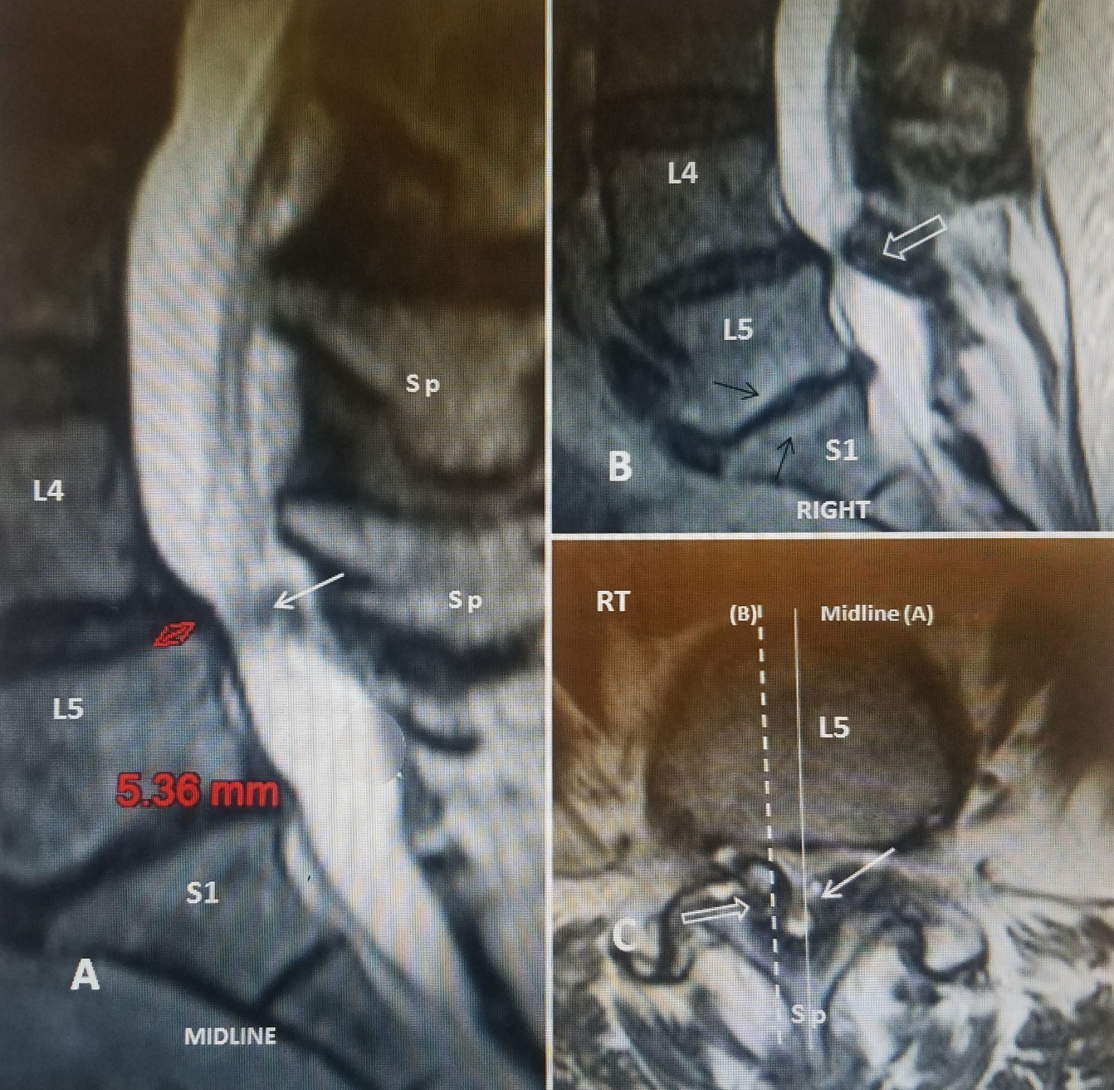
Magnetic resonance imaging demonstrating how to correlate sagittal to axial imaging in lumbar spinal stenosis. A: Midline T2 sagittal slice shows grade 1 spondylolisthesis at L4-5 (solid double-headed red arrow) with a anterior-posterior canal dimension of 5.36 mm. The moderate stenosis seen on this midline slice, identifying by the spinous processes (sp) shows the compression at L4-5 is primarily posteriorly (solid white arrow). B: Sagittal T2 slice made slightly more lateral than the cut in 'A'. The posterior stenosis (open white arrow) now appears more severe than in 'A'. C: Axial T2 MRI in the same patient indicates where each slice is located and the right sided indicated (RT). The midline slice (solid thin white line) including the spinous process (sp), corresponds to 'A'. This is indicated that 'A' is adjacent to the enlarged facet bone and joint (solid white arrow). The more right sided cut (dashed white line) corresponding to 'B' shows the markedly enlarged facet joint and thickened joint capsule and ligament (open white arrow) corresponding to the right lateral recess. In this patient, correlating axial and sagittal MRI scan slices demonstrates the stenosis is worse in the lateral recesses than the midline.
